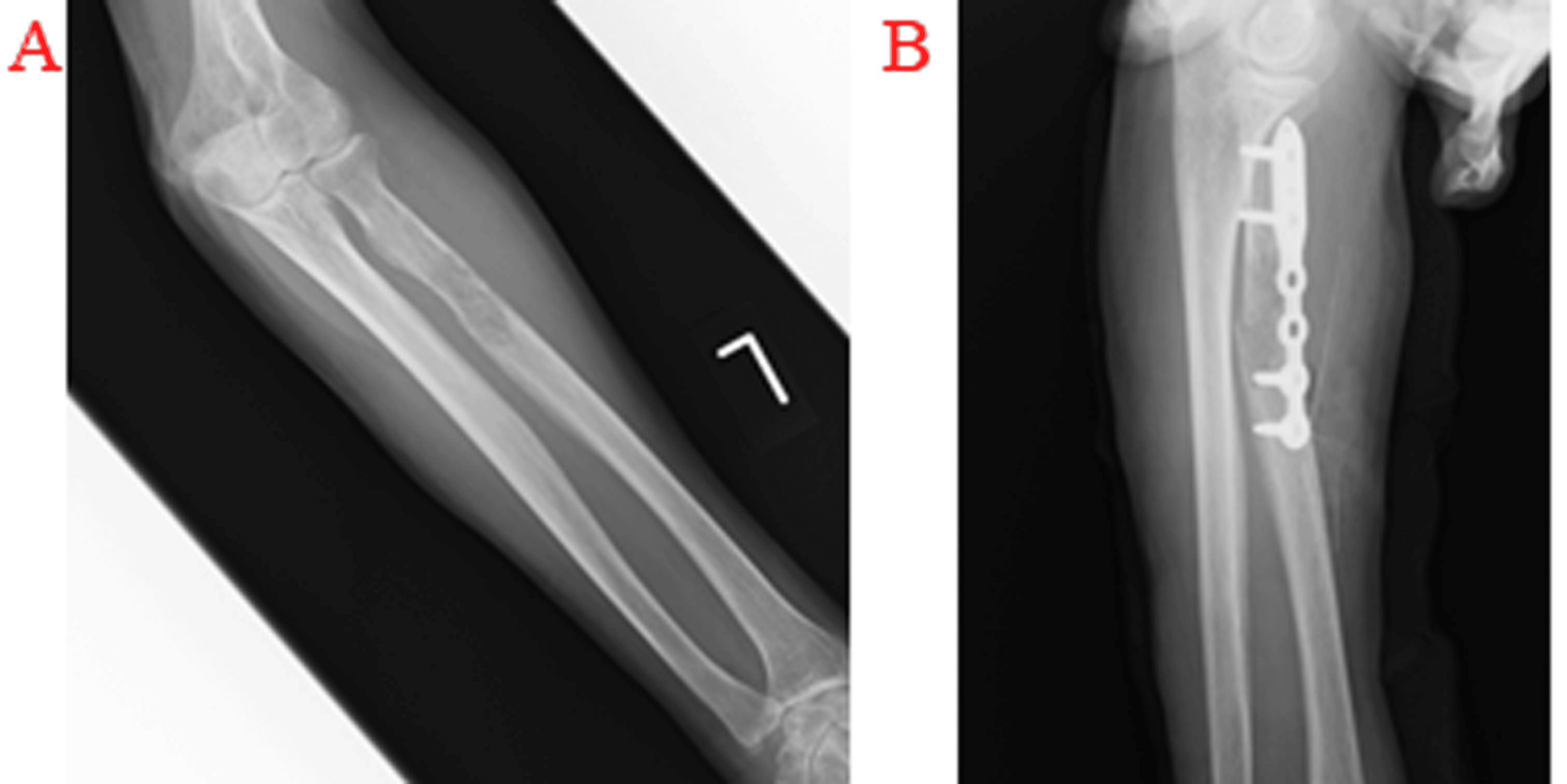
Radiographic findings of a pathological fracture of the proximal left radius. (A) The primary lesion was plasmacytoma with osteolysis. (B) Radiographic findings after fixation of the lesion with a plate.
